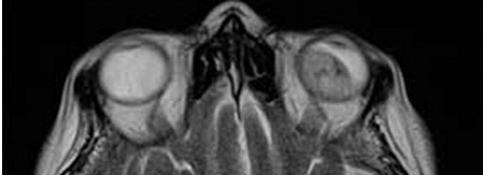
Choroidal malignant melanoma - magnetic resonance imaging
